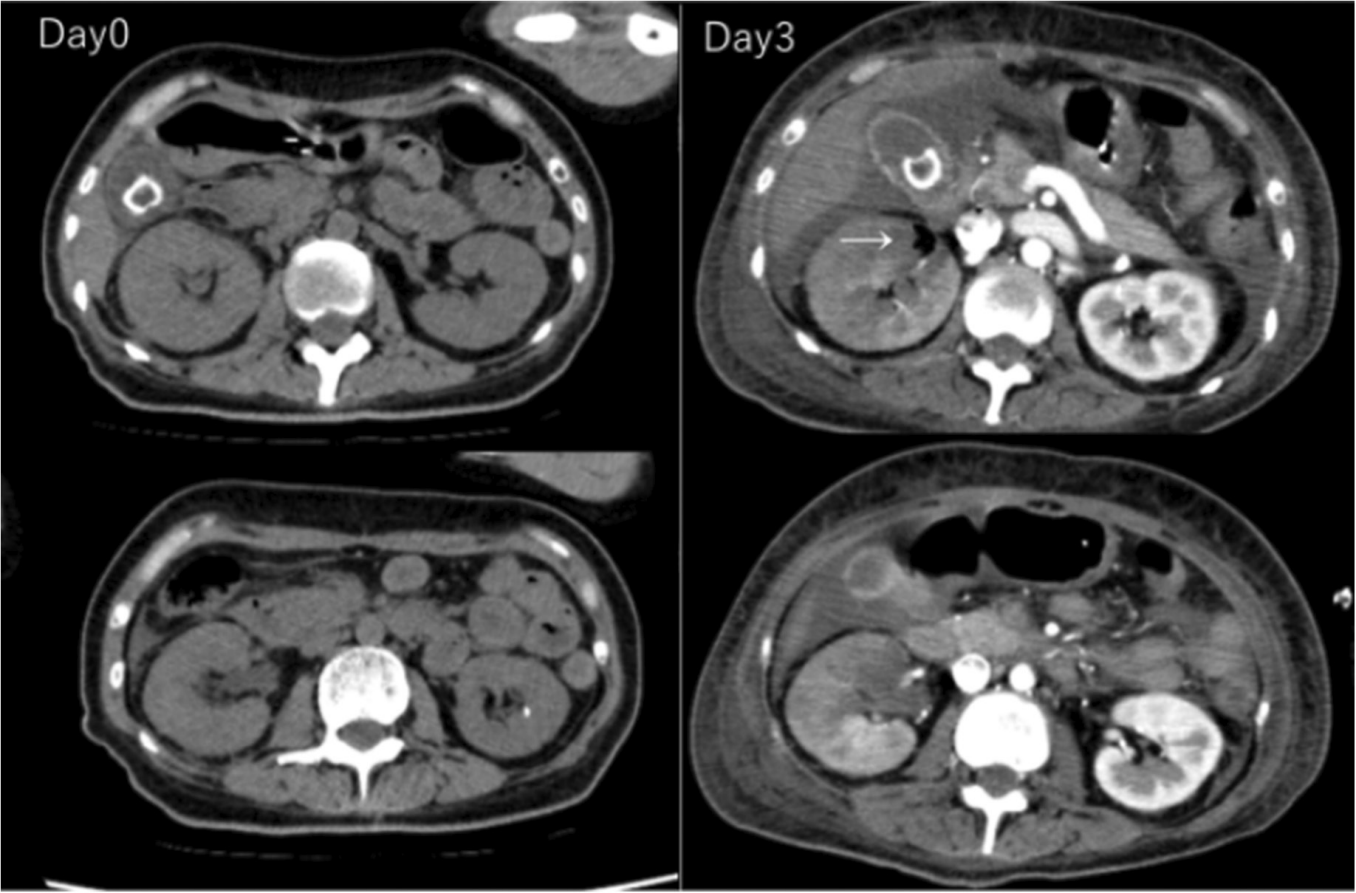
Computed tomography (CT) imaging results on the 0th and 3rd day of hospitalization. On the 3rd day of hospitalization, new renal emphysema formation was observed in the right kidney (white arrow).
